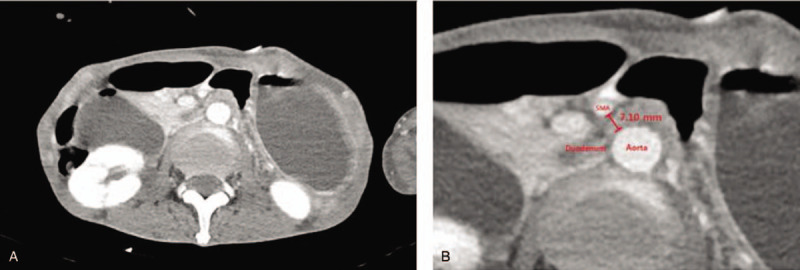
Abdominal CT findings at the time of diagnosis. (A) The second portion of the duodenum narrowed abruptly at the site between the aorta and the superior mesenteric artery. Near obstruction and severe dilatation of the stomach and proximal duodenum were observed. (B) The distance between the SMA and aorta was 6.23 mm, which is shorter than the normal range. CT = computed tomography, SMA = superior mesenteric artery.
